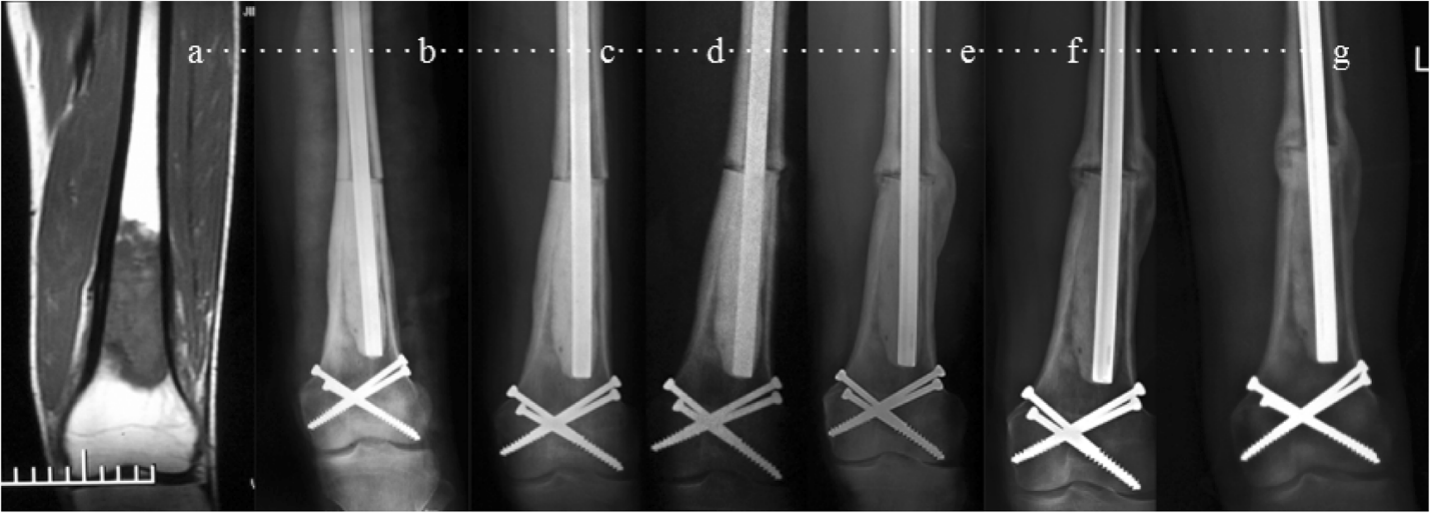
A 20-year-old male patient with osteosarcoma in left distal femur was treated with alcohol-inactivated autograft replantation with articulation preservation. a Preoperative MRI showed intramedullary low mixed signal in T1 in distal femur. The lowest boarding of tumor lies 2cm over Insall line which was classified as Type I. b-d Postoperative X-ray at 1 week, 3 months and 6 months. e and f 16 months and 20 monthes after operation, X-ray showed bone callus in diaphysis, and bony healing in conjunction between host bone and inactivated bone. g 35 months after operation, X-ray showed fully bony healing and good joint space. At the end of follow-up, he has returned to normal work with 33 in MSTS score and 53 in QOL.
